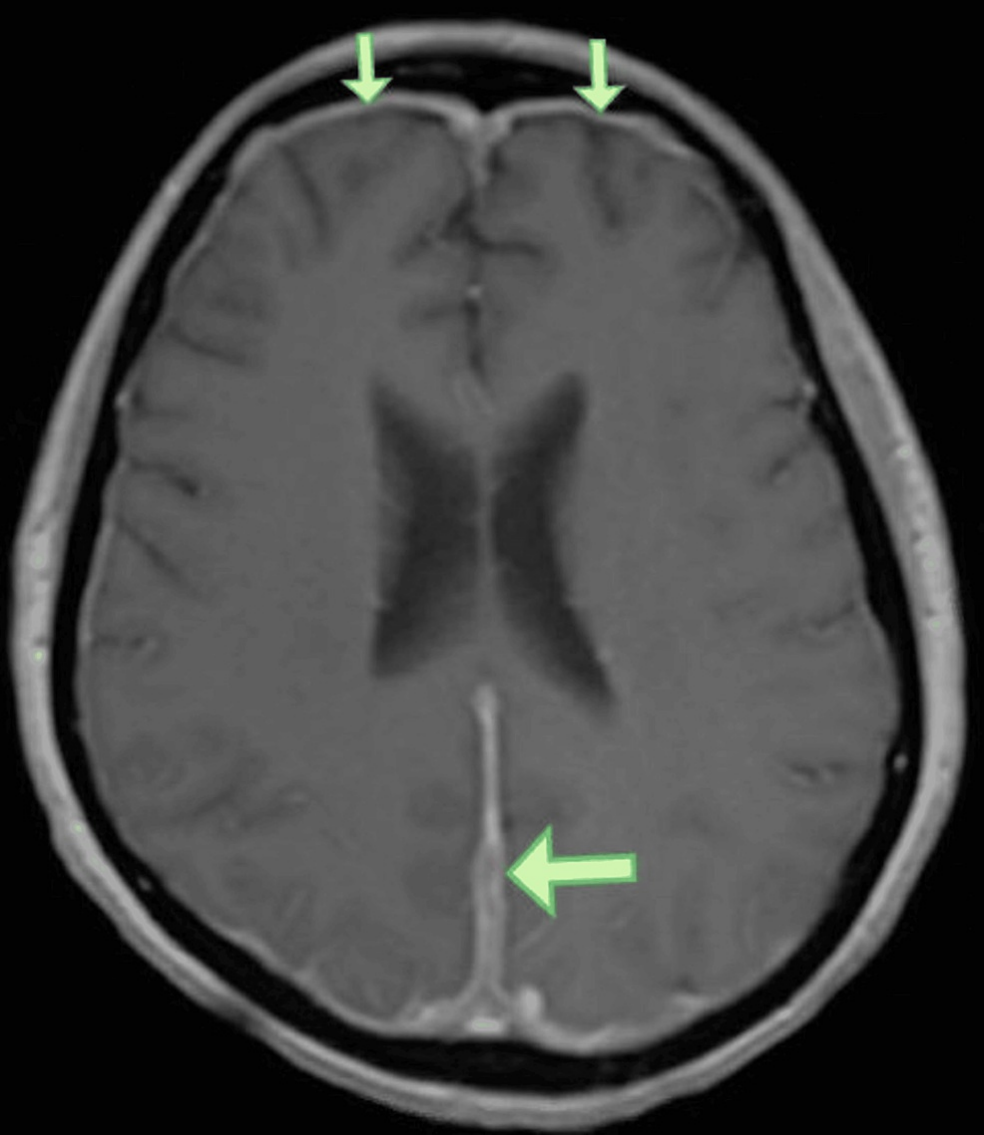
MRI of brain w/wo gadolinium contrast, T1 sagittal view Green arrows pointing toward the contrast enhancement of the meninges.
